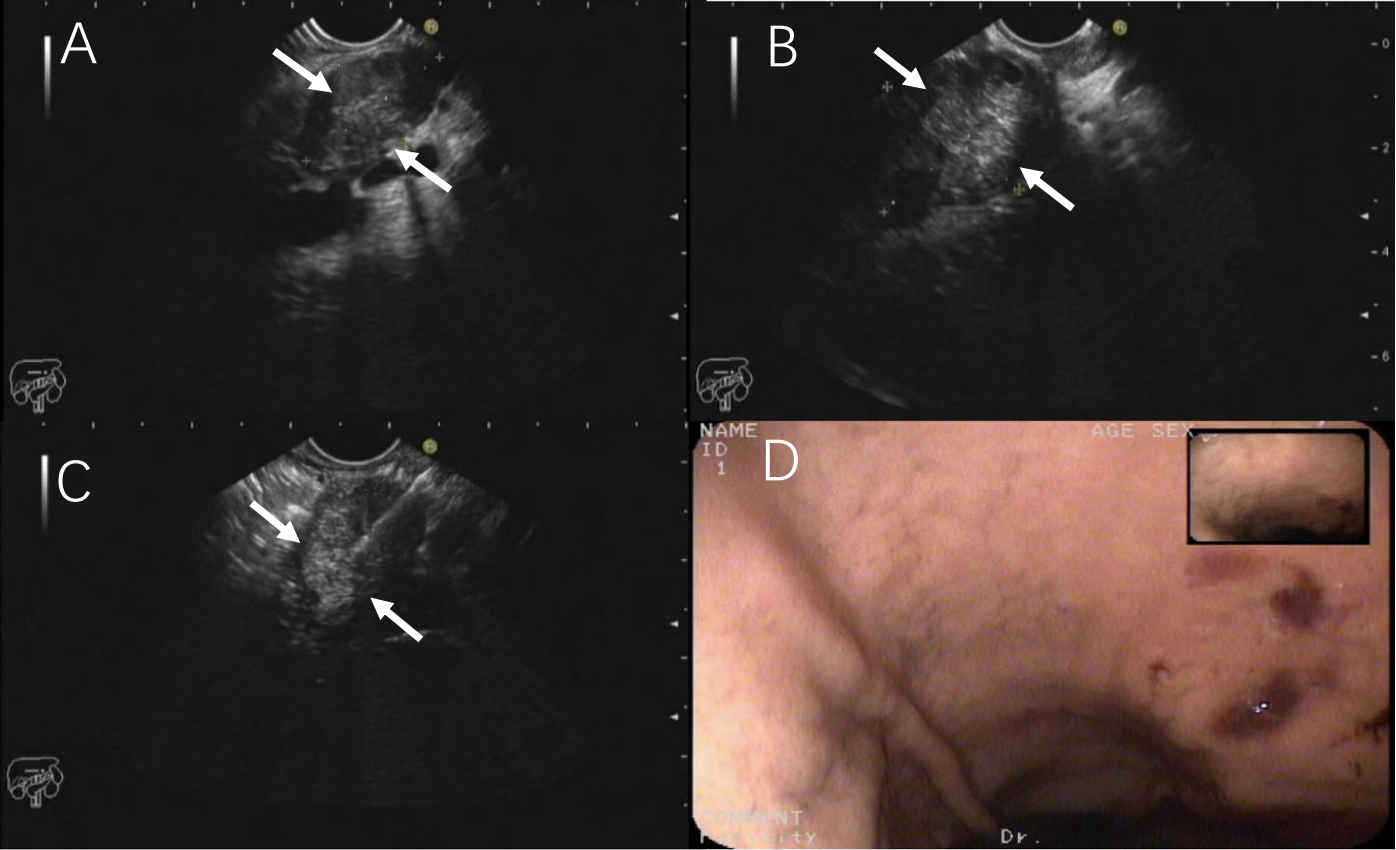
Endoscopic ultrasound showed diffuse enlargement of the pancreas, dilated intra-/extra-hepatic bile duct, and enlarged gallbladder; occupancy of the head of the pancreas was suspected
